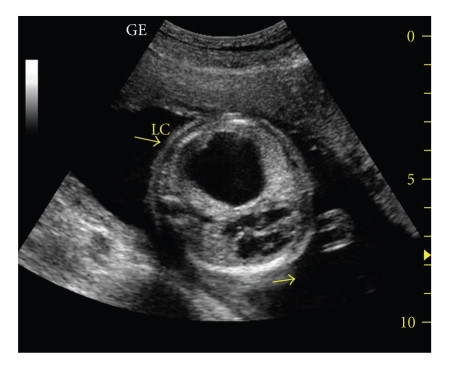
Sonographic examination revealed uniloculated, 40 × 37 mm sized, smooth contour congenital cystic lung lesion which originated from left hemi thorax.
